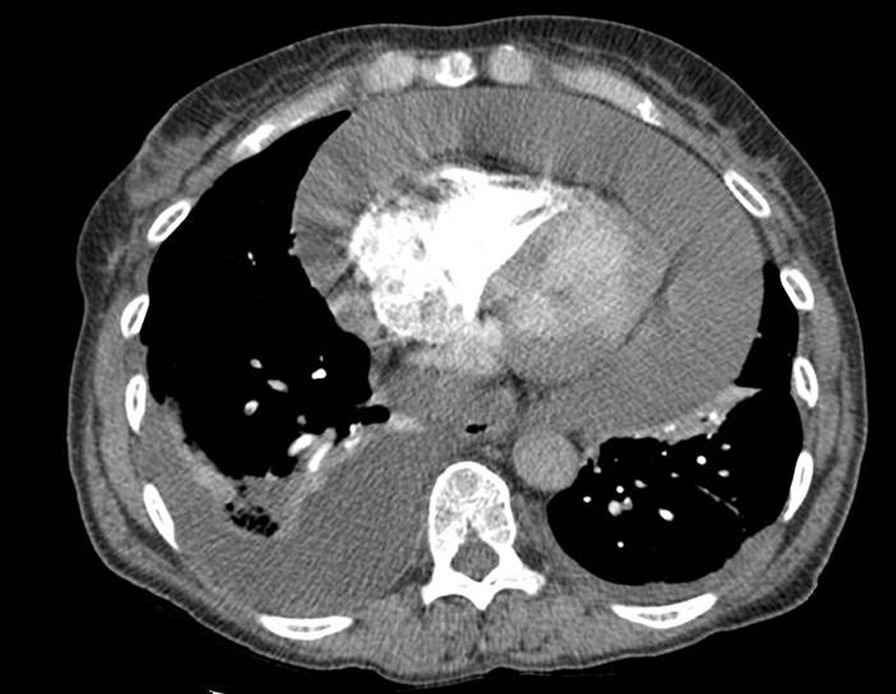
Computed tomography scan showing very large pericardial effusion and possibly reflecting the presence of cardiac tamponade. There is moderate right posterior pleural effusion
